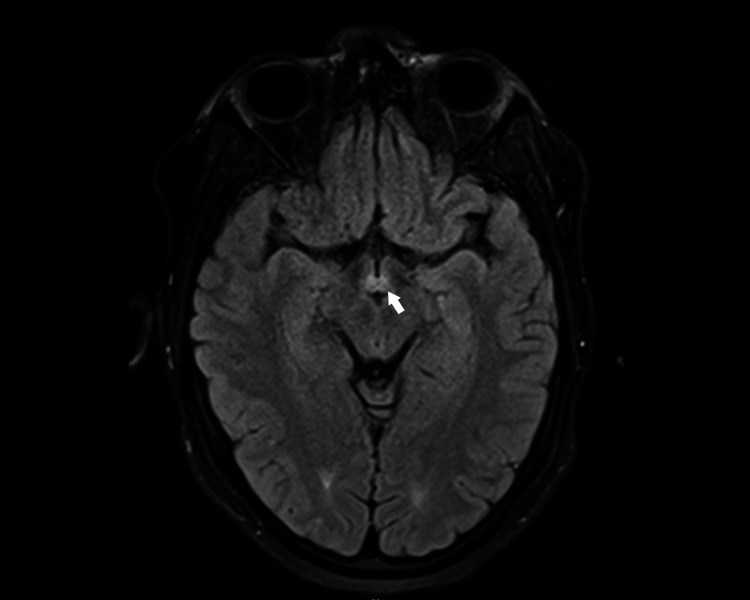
MRI brain White arrow: axial T2 flair showing T2 hyperintensity of the mammillary bodies bilaterally
